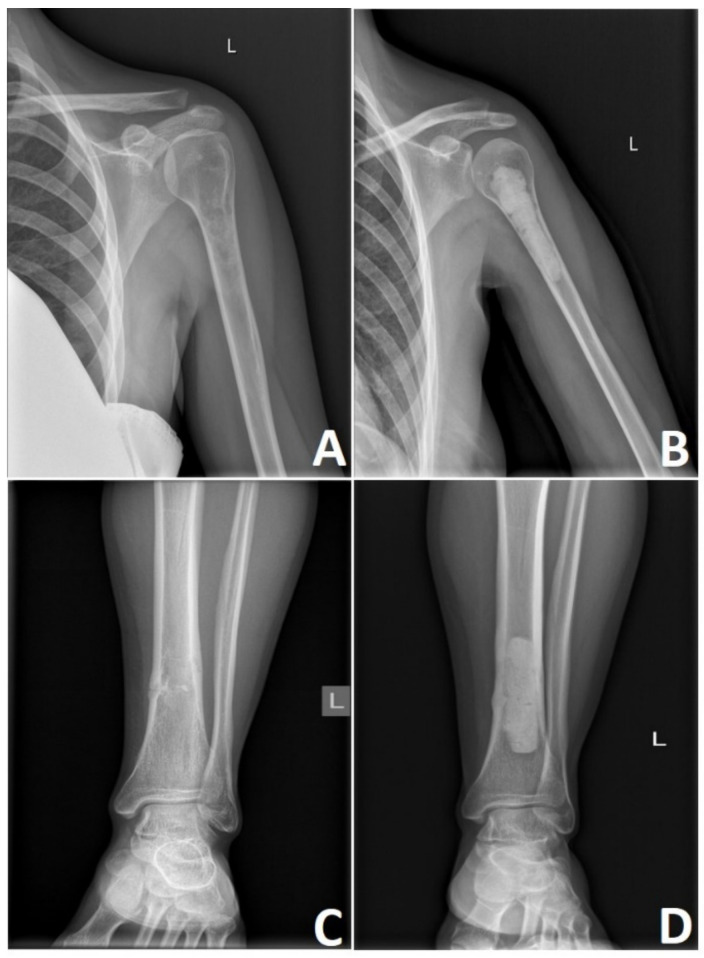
Low-grade chondrosarcoma. Rarely symptomatic lesions are discovered incidentally on X-rays after injury to the extremity (A,C). Meticulous curettage of G1 chondrosarcomas and polymethyl methacrylate cementoplasty remains the mainstay of treatment (B,D). Photos by Bartłomiej Szostakowski.
